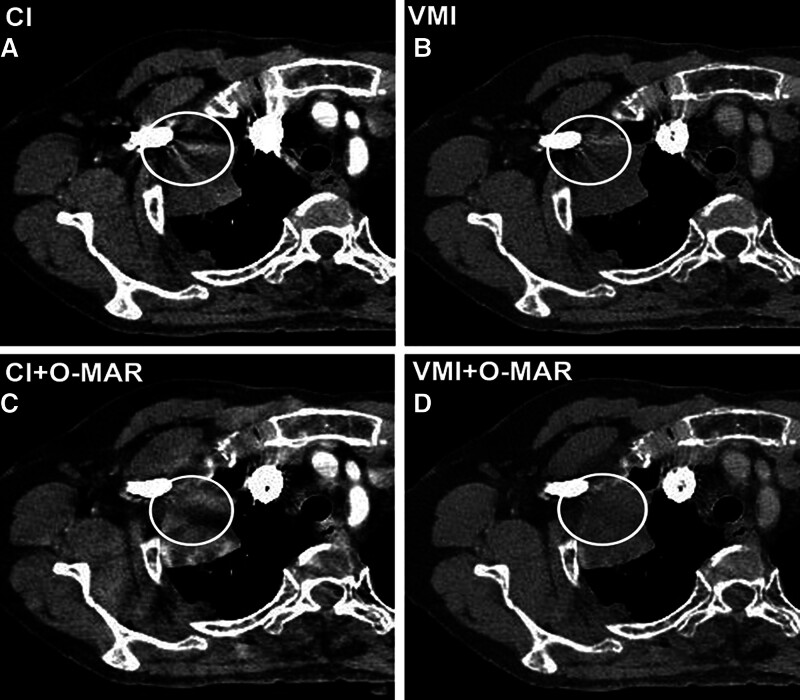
An 83-year-old woman with a soft tissue mass in the right upper lobe. Enhanced chest CT images show a soft tissue mass with irregular margin and homogeneous density (circles). On CI (A), it is difficult to assess the mass because of the severe artifacts due to CM in the subclavian vein. VMI (B) reduces image artifacts to some extent, but some remaining artifacts are still visible. The CI + O-MAR image (C) reduces the artifacts, but some new streak artifacts are observed. However, the VMI + O-MAR image (D) reduces most of the artifacts and enables the evaluation of the shape, margin, and density of the mass. CI = conventional images, CM = contrast media, O-MAR = orthopedic metal-artifact-reduction, VMI = virtual monoenergetic images.
